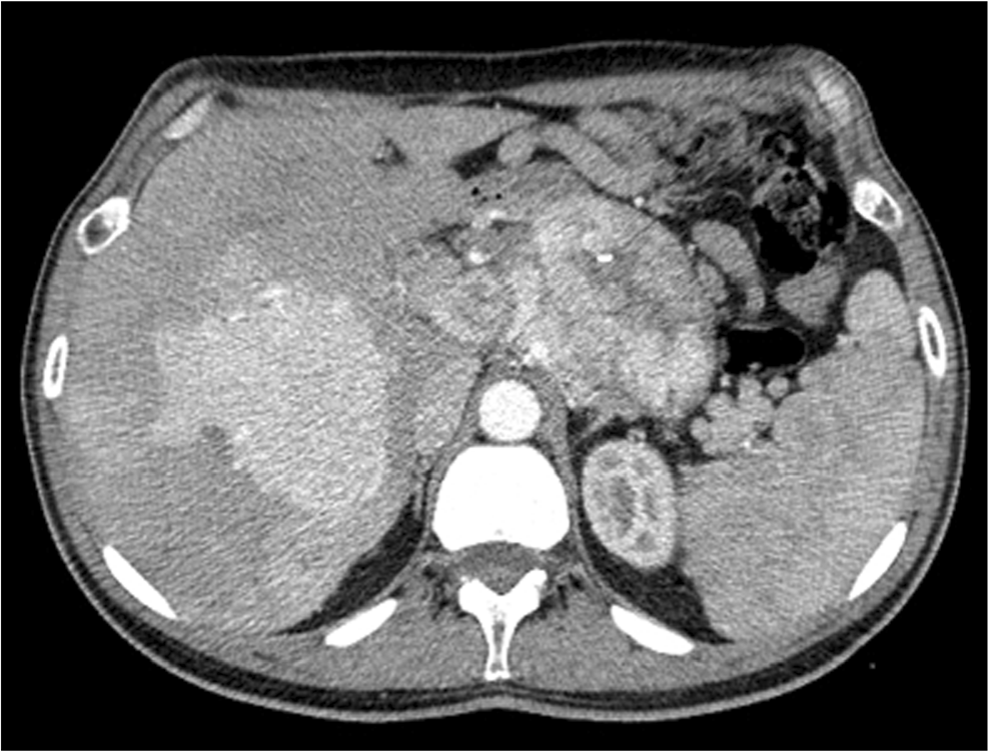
Non-functioning NEN. CT examination: huge inhomogeneously hypervascular pancreatic body-tail mass with necrotic areas and intralesional calcifications. Liver metastatic involvement is present
